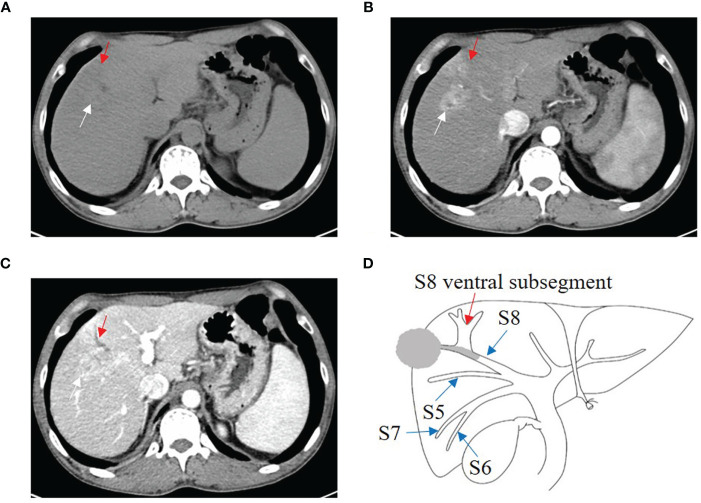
HCC with B1 BDTT. (A) The HCC lesion (white arrow) and bile duct dilation in S8 (red arrow) show hypoattenuation in plain CT image. (B) The HCC lesion has early enhancement (white arrow) in arterial phase, but enhancement of the bile duct dilation (red arrow) was not observed. (C) The HCC lesion (white arrow) shows hypoattenuation at portal venous phase, accompanied by dorsal bile duct dilation (red arrow) in S8. (D) HCC lesion, BDTT, and bile duct dilation (red arrow).
